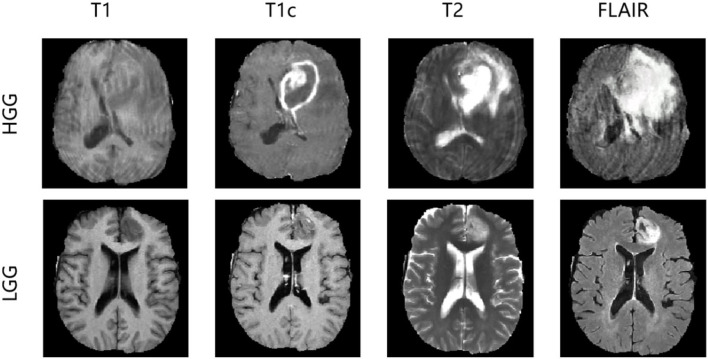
HGG and LGG cases with four modalities: T1, T1c, T2, FLAIR on BRATS2015 dataset.
